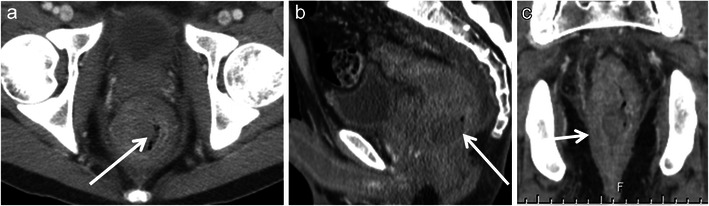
Rectal Kaposi’s sarcoma in a 29-year-old HIV-positive man. a Axial, b sagittal and c coronal CT reveal nodular submucosal mass (*arrow*) in the rectum with rectal wall thickening
